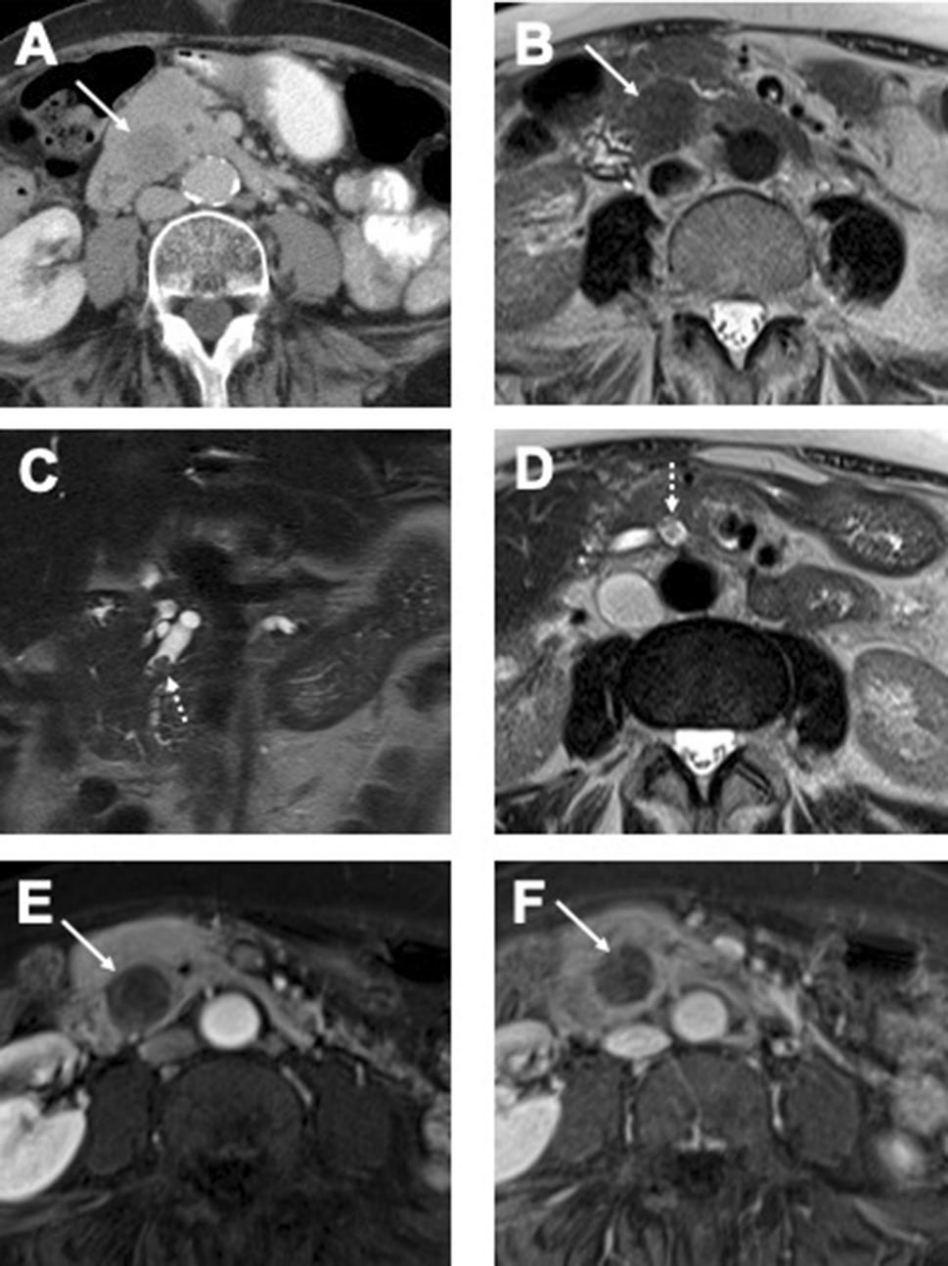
CT and MRI features of ITPN with intraductal solid tumor growth. (A) Axial CT scan in the portal venous phase shows an ITPN lesion in the pancreatic head (arrow) with well-circumscribed margins and slightly hypodense HU-values, compared to normal pancreatic parenchyma. (B) Axial T2 weighted image of the same patient. The lesion had T2 hypointense signal (arrow), suggesting a solid morphology. (C,D) Coronal T2 weighted image (C) and axial T2 weighted image (D) show dilated main pancreatic duct with intraductal solid tumor growth (dotted arrows). (E,F) T1 weighted images with fat suppression after contrast administration in the arterial (E) and venous (F) phase. In both phases tumor is hypointense to pancreatic parenchyma without significant contrast enhancement.
